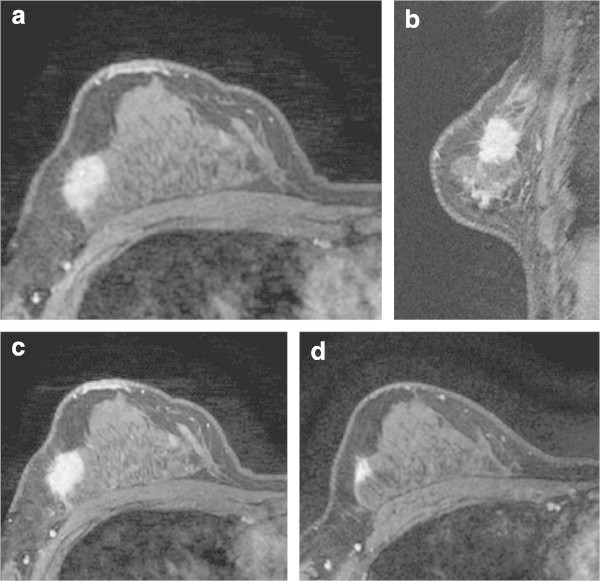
Luminal A breast cancer of right breast in 40-year-old woman. Prior to NAC, dynamic MR images obtained at 60 s (a), 240 s (b), and 300 s (c) after administration of gadolinium show a persistent enhancing mass with an irregular border. After the completion of NAC, residual tumor still shows an enhancement on dynamic MR image obtained at 60 s (d).
